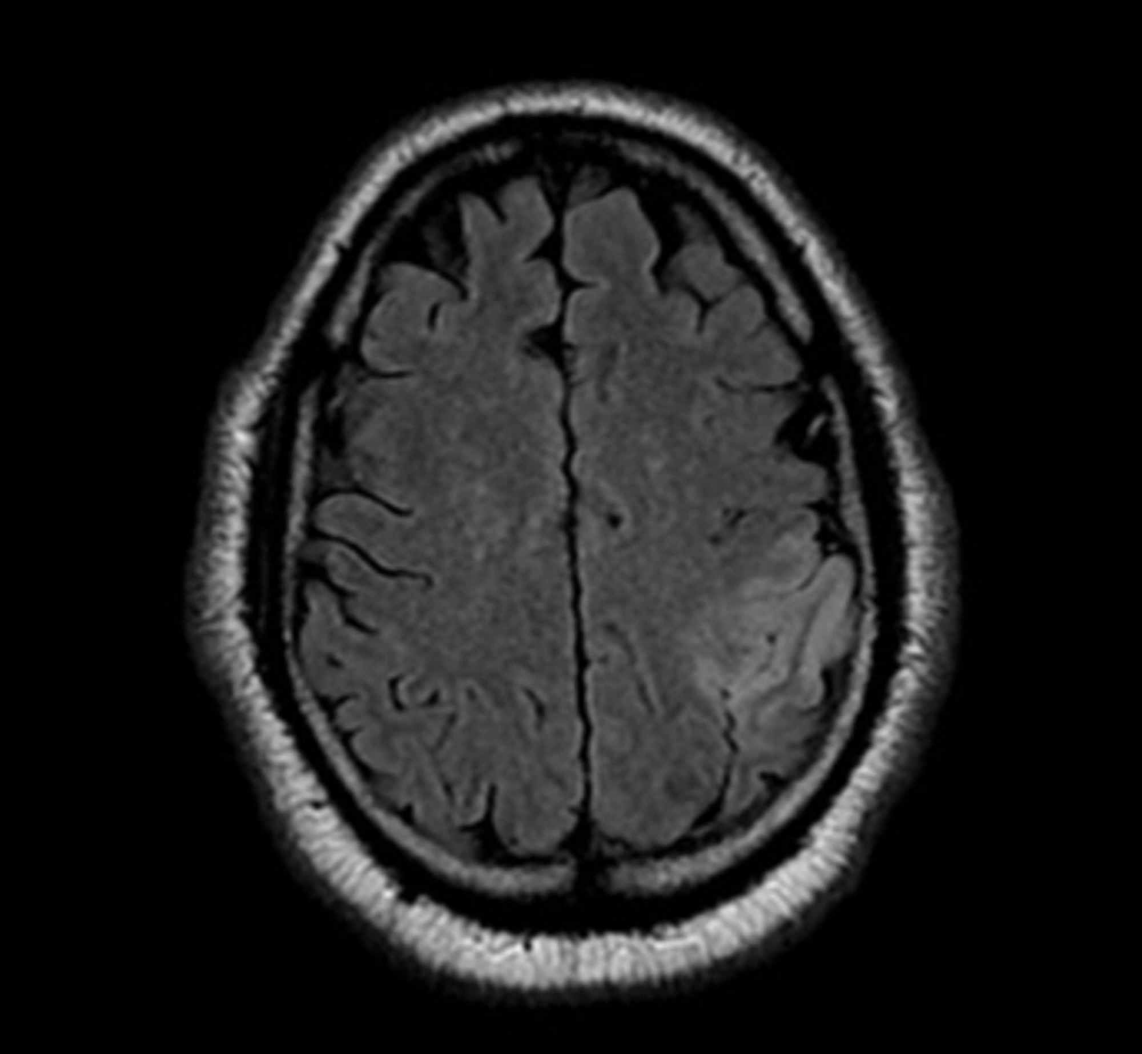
MRI of the brain without contrast: Axial T2 flair There is an area of restricted diffusion seen in the left inferior parietal lobe, including the postcentral gyrus, as well as associated increased signal intensity on FLAIR consistent with acute ischemic insult in left middle cerebral artery (MCA) vascular territory.
